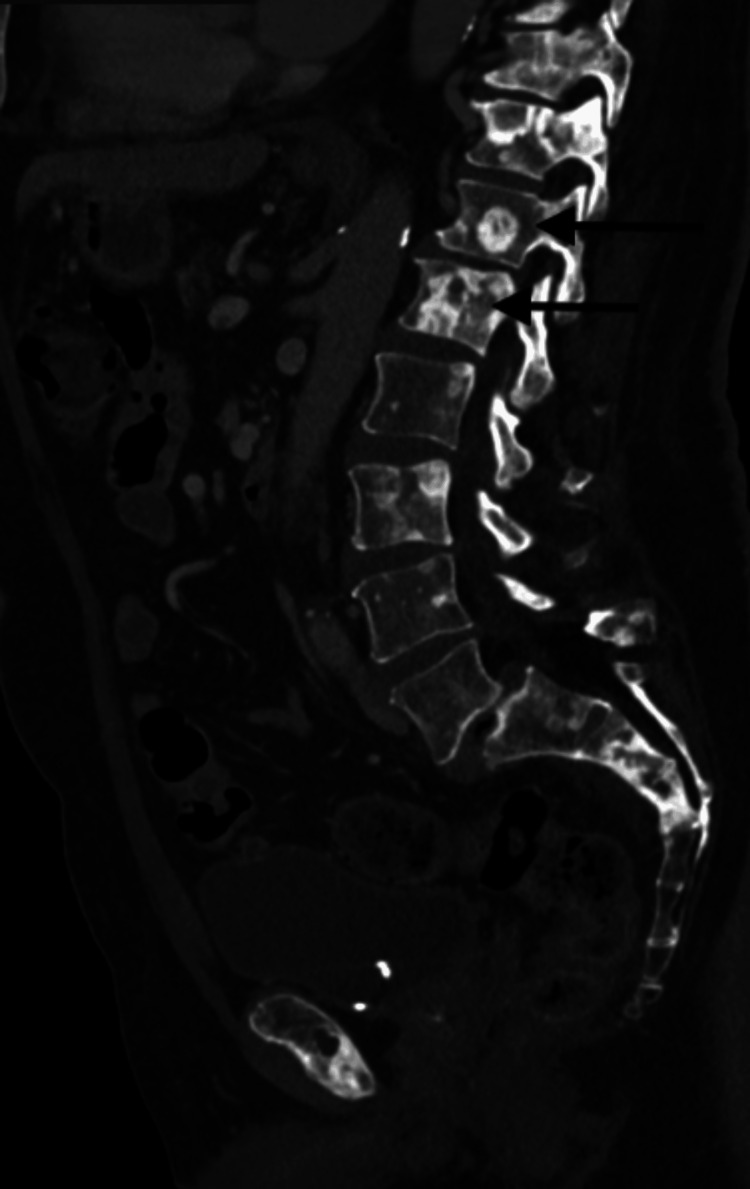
CT showing multiple osseous metastases in the sagittal view
